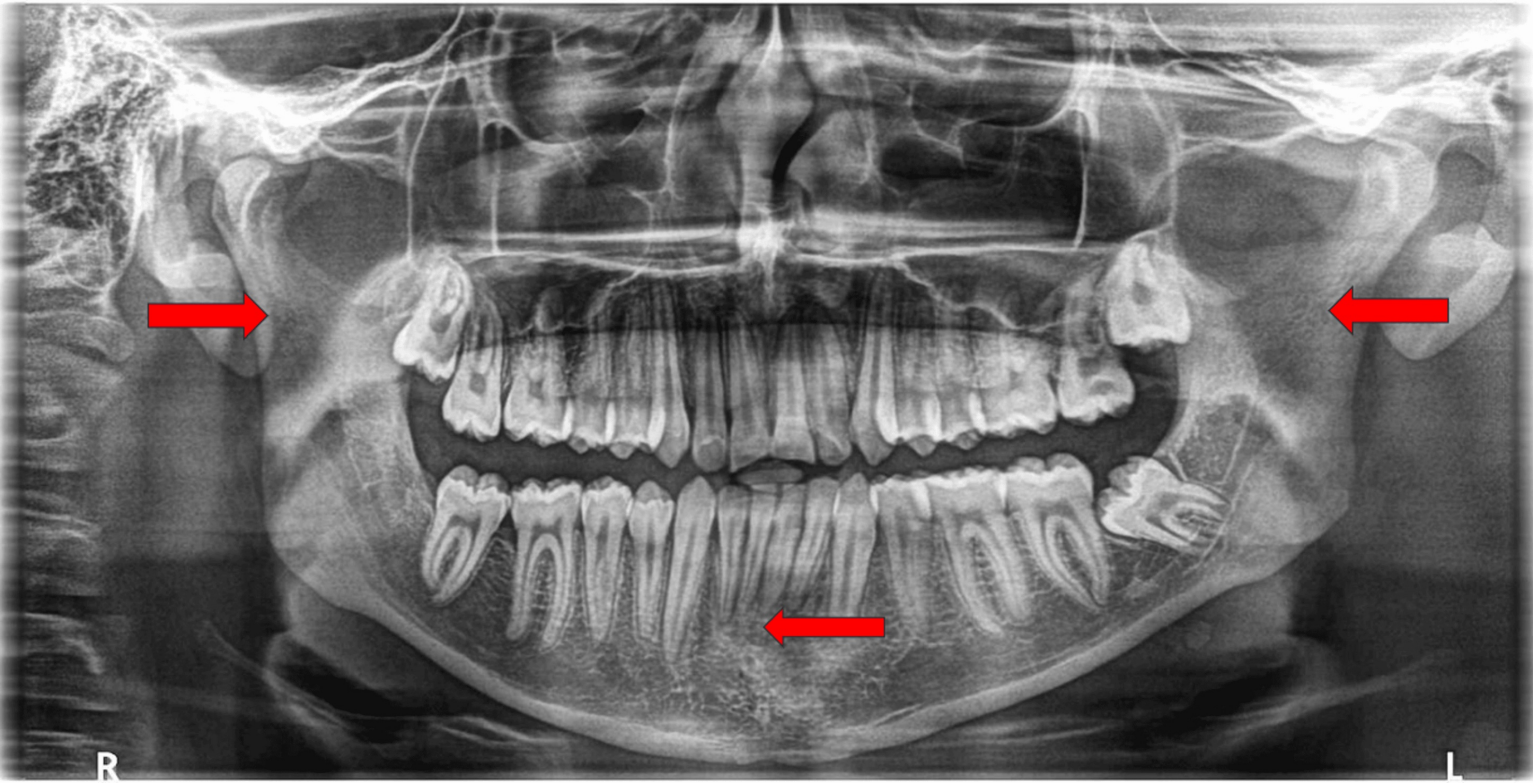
Nine-month postoperative orthopantomogram (OPG) showing bony union of the fractured mandible (red arrows).
